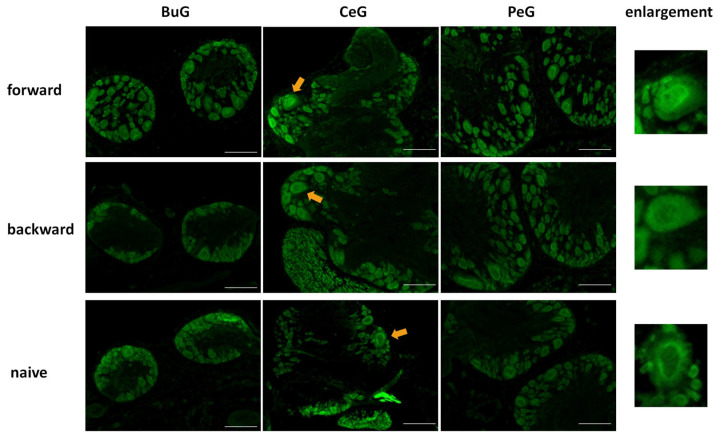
LymFOXO localization changes in the CNS sections of food-satiated Day −1 snails after CTA conditioning. BuG: buccal ganglion. CeG: cerebral ganglion. PeG: pedal ganglion. Green indicates LymFOXO immunoreactivity. Arrows indicate enlarged neurons shown in the right panels. The enlarged figure of a neuron from the CNS of a forward-conditioned snail shows that LymFOXO is present in the nucleus, whereas those from backward-trained and naive control snails show that LymFOXO is present in the cytoplasm. Scale bars: 100 µm.
